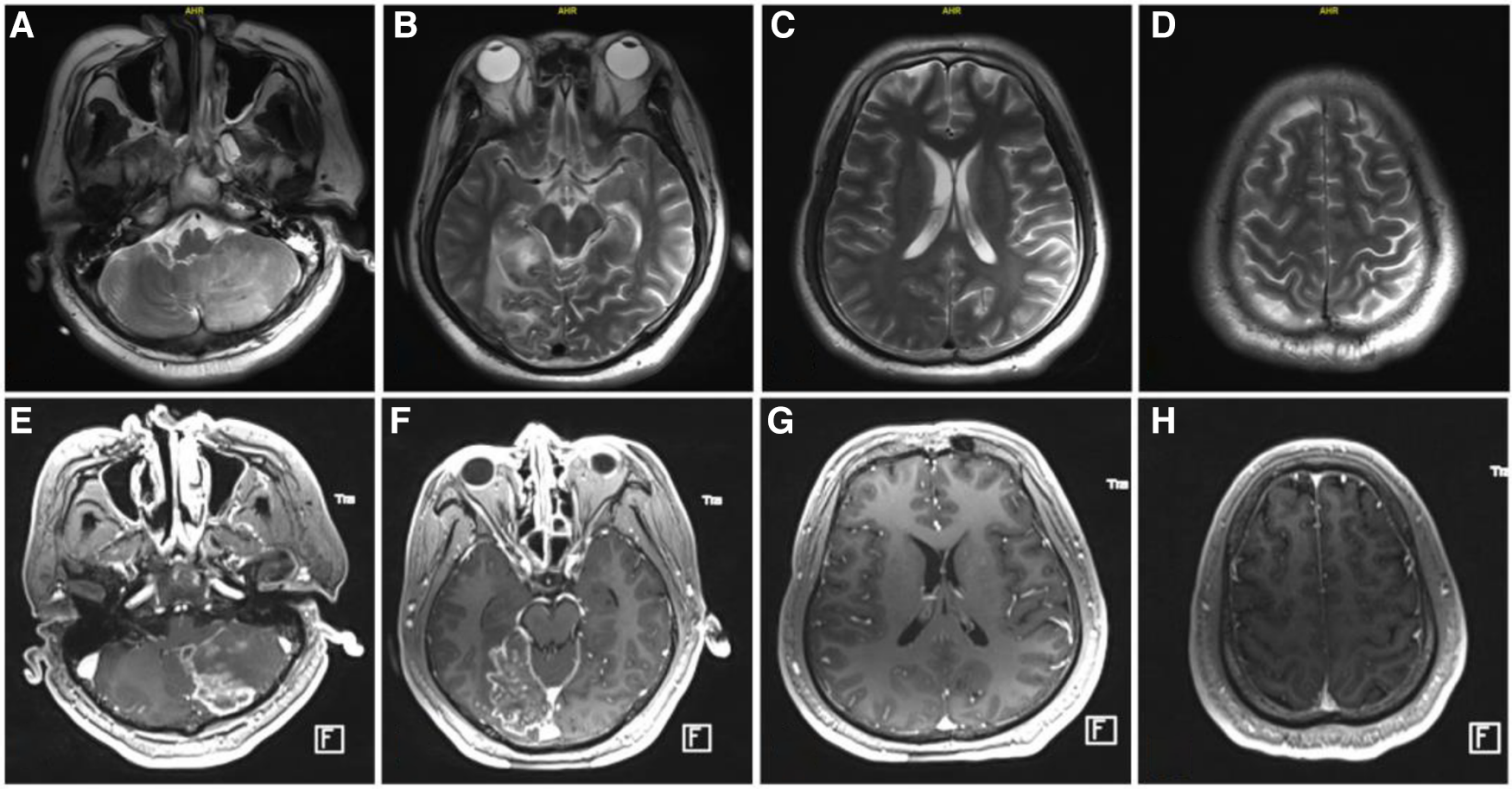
Cranial MRI at day 11 after admission. (A–D), MRI plain scan; (E–H), enhanced MRI.
